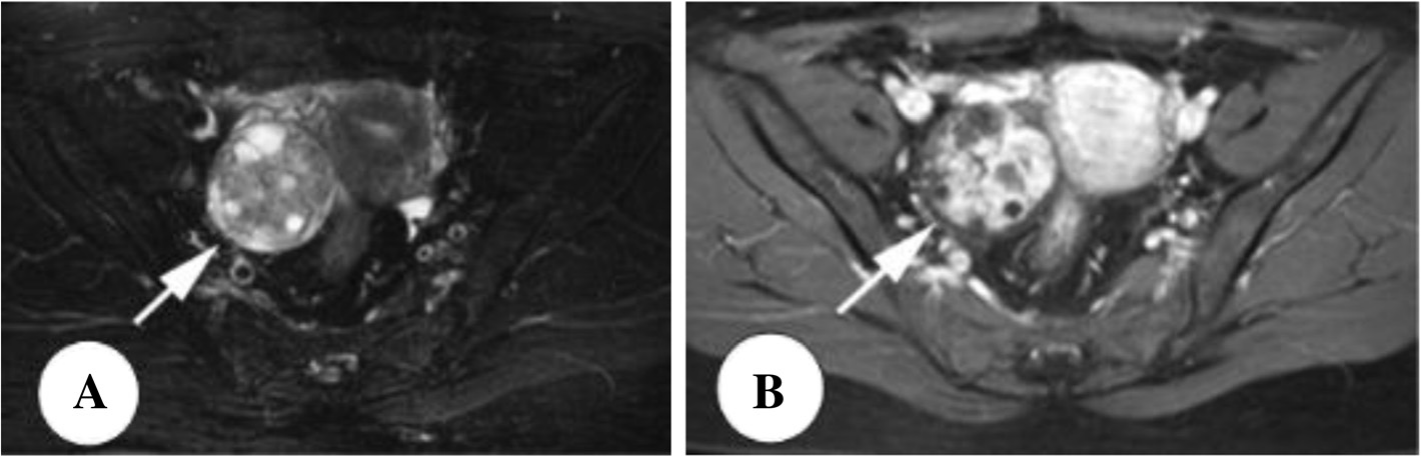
Sertoli-Leydig cell tumor in a 46-year-old woman without symptoms. (A) Axial T2-weighted image with fat saturation shows a solid mass with multiple small cysts (arrow). (B) The solid components are the moderate signal intensity and obviously enhanced after the administration of contrast medium.
